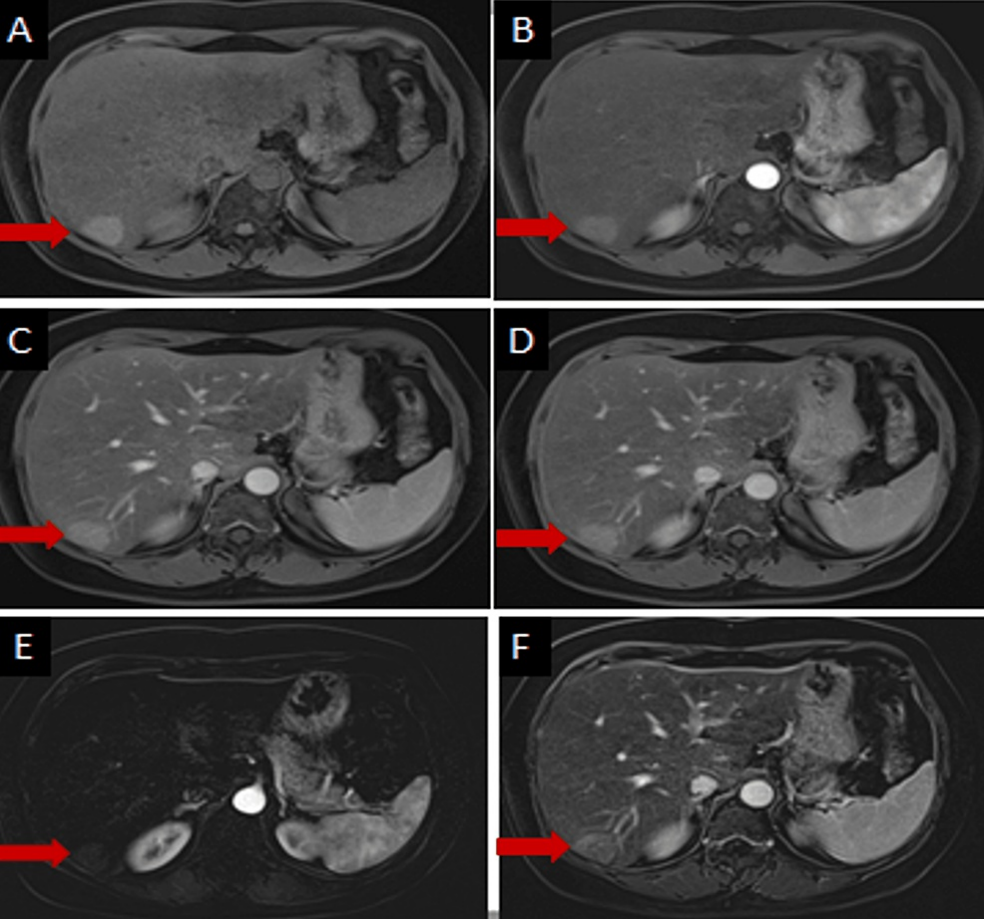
Dynamic gadolinium-enhanced T1-weighted images (A: precontrast, B: arterial, C: venous, D: delayed phases), The lesion in segment 6 appears relatively hyperintense on the precontrast fat-suppressed image (red arrow in A) with suppression of fatty signal intensity of the surrounding liver parenchyma. This lesion appears homogenously hyperintense on all three post-contrast phases with no washout relative to liver parenchyma (red arrows in B-D). Subtraction images (A: arterial & F: Venous) show mild homogenous enhancement and no washout.
